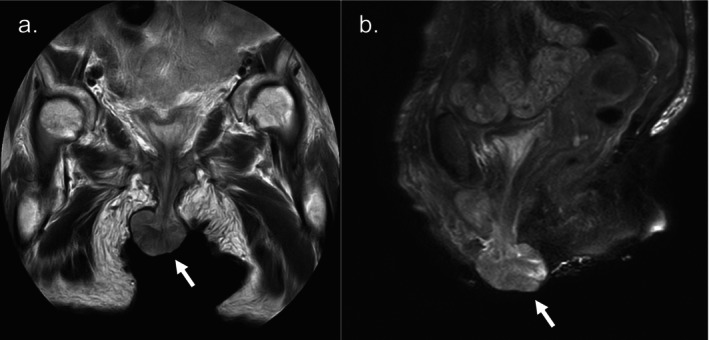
Magnetic Resonance Imaging images: A tumor continuous with the urethra shows mildly high signal intensity on T1‐weighted imaging and high signal intensity with internal striations on T2‐weighted imaging.
